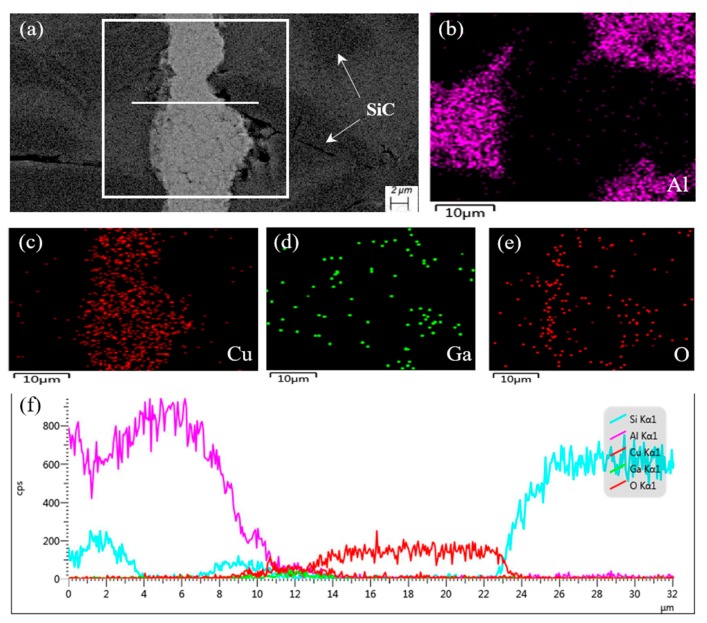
SEM image of typical region at the interface of the 65 vol.% SiC_p_/6063 Al MMC joint made at 450 °C and corresponding energy dispersive X-ray maps showing distribution of elements: (a) SEM image; (b–e) individual elemental mapping of Al, Cu, Ga, and O, respectively; (f) line scanning profile.
